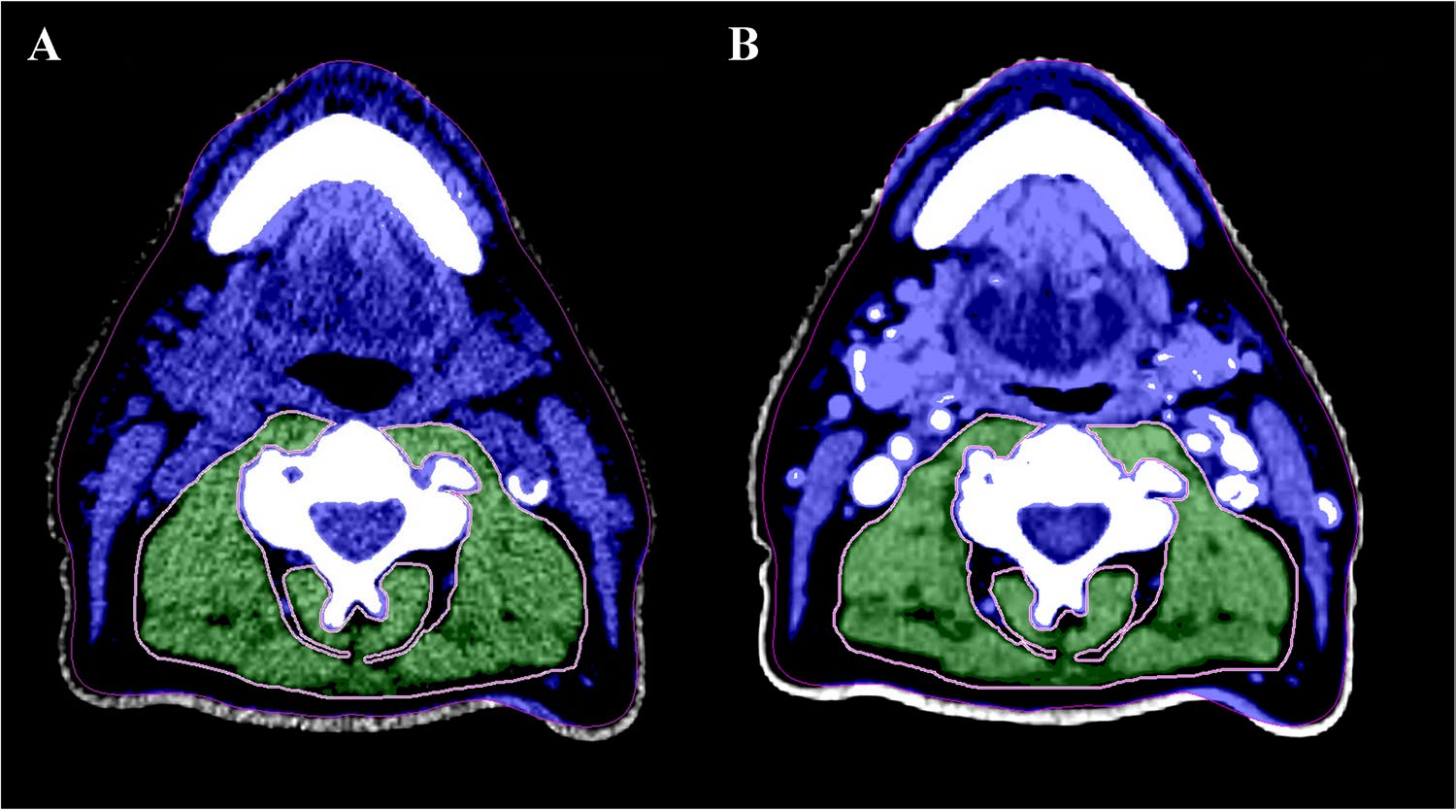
Delineation of paravertebral muscle in green at C3 level made on LD from the [^18^F]-FDG PET-CT scan (A) and diagnostic neck (B) CT scan. Blue and green are within the skeletal muscle HU thresholds. Additionally, both sternocleidomastoid muscles were delineated to calculate the CSA at C3 level. C3, third cervical vertebra; CSA*,* cross-sectional area; CT, computed tomography; LD, low dose
